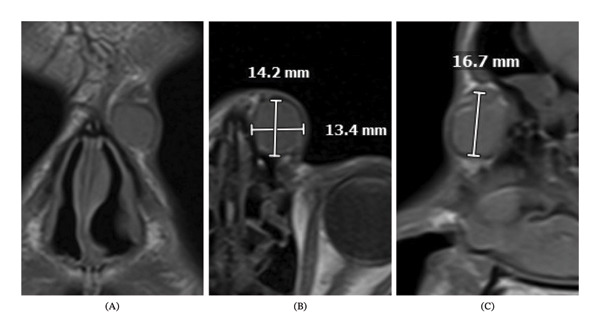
T1‐weighted MRI images: (A) coronal view, (B) axial view, and (C) sagittal view of the patient.
